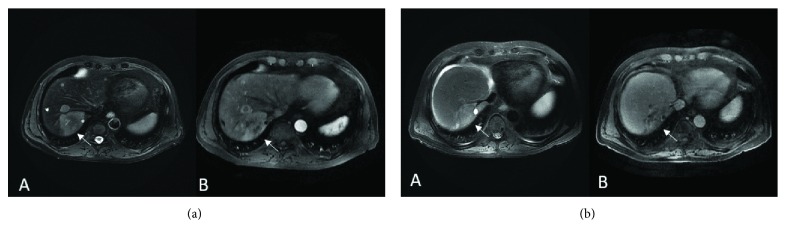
(a) Pretreatment scans (MRI). Axial T2 WI (A) and postcontrast (Eovist) Axial T1 WI (B) showing a 7 cm mass in the right liver lobe (arrows) and multiple smaller lesions in both liver lobes. (b) Posttreatment scans (MRI). Axial T2 WI (A) and postcontrast (Eovist) Axial T1 WI (B) show the decrease in size of the largest mass in the right liver lobe (arrows).
